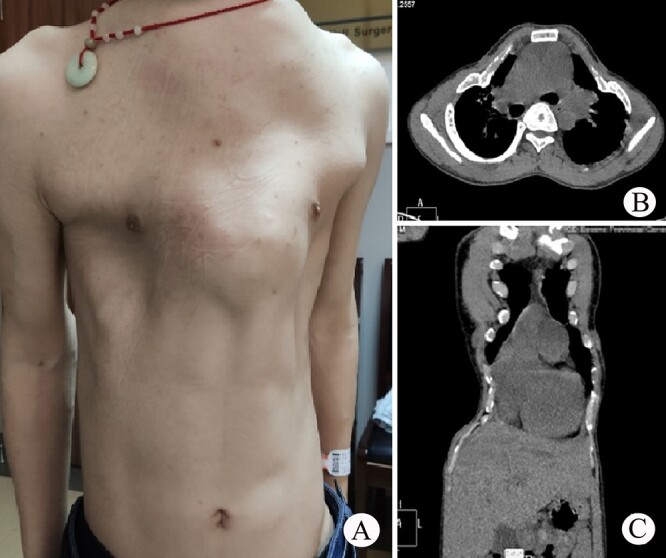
(A) Thorax appearance before operation; and (B) and (C) CT scan of thorax.
